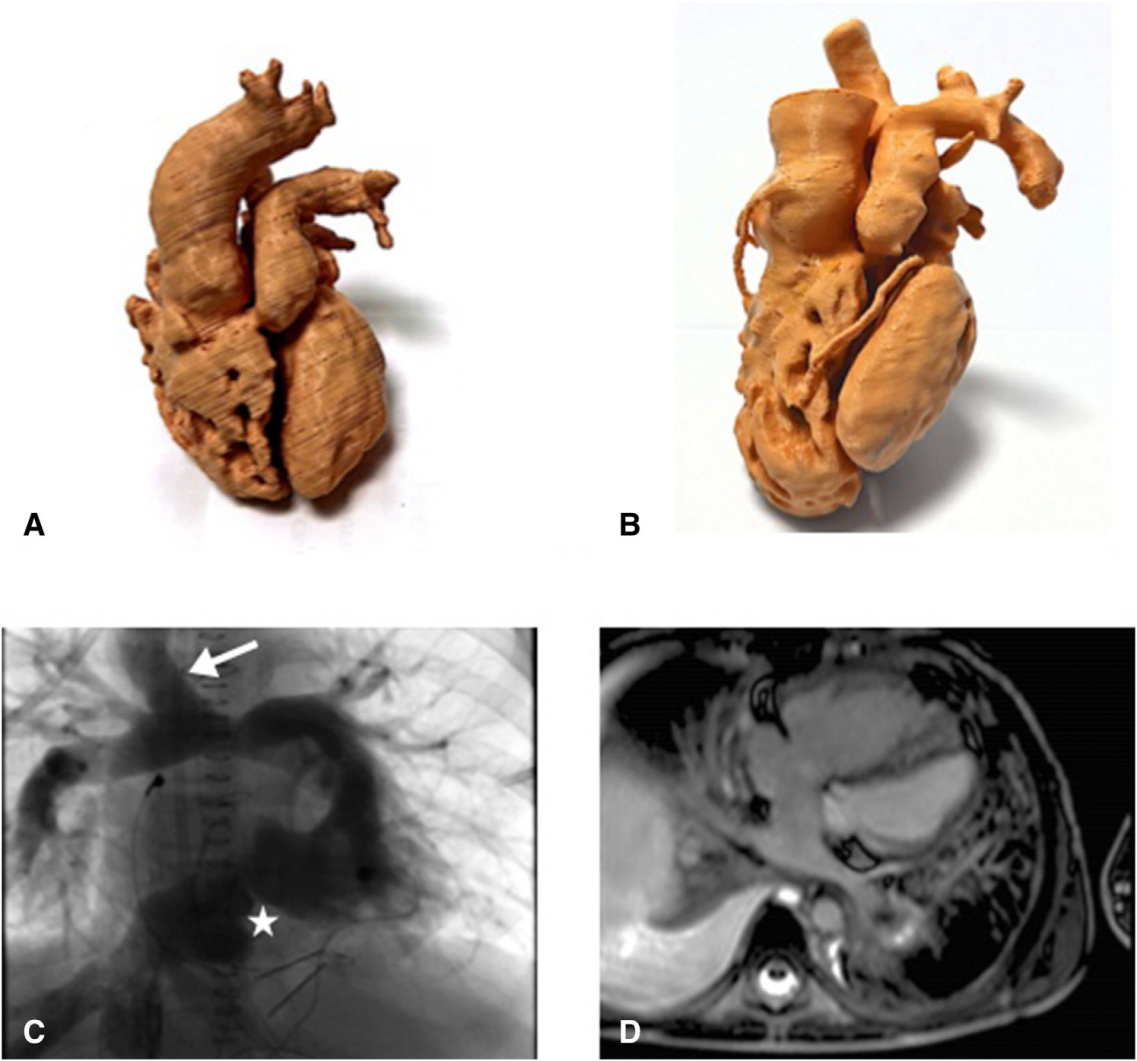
A, Three-dimensional (*3D*) cardiac silicone model, constructed during infancy, showing that both ventricles were well developed. B, 3D cardiac silicone model constructed at the age of 10 years, showing that the left ventricle is noticeably smaller than the right ventricle. C, Angiogram showing flow reversal through the superior cavopulmonary connection (*arrow*) and a narrow hemi-Mustard pathway (*star*). D, Cardiac magnetic resonance imaging, done after the ventricular switch procedure, showing 2 ventricles of normal size.
